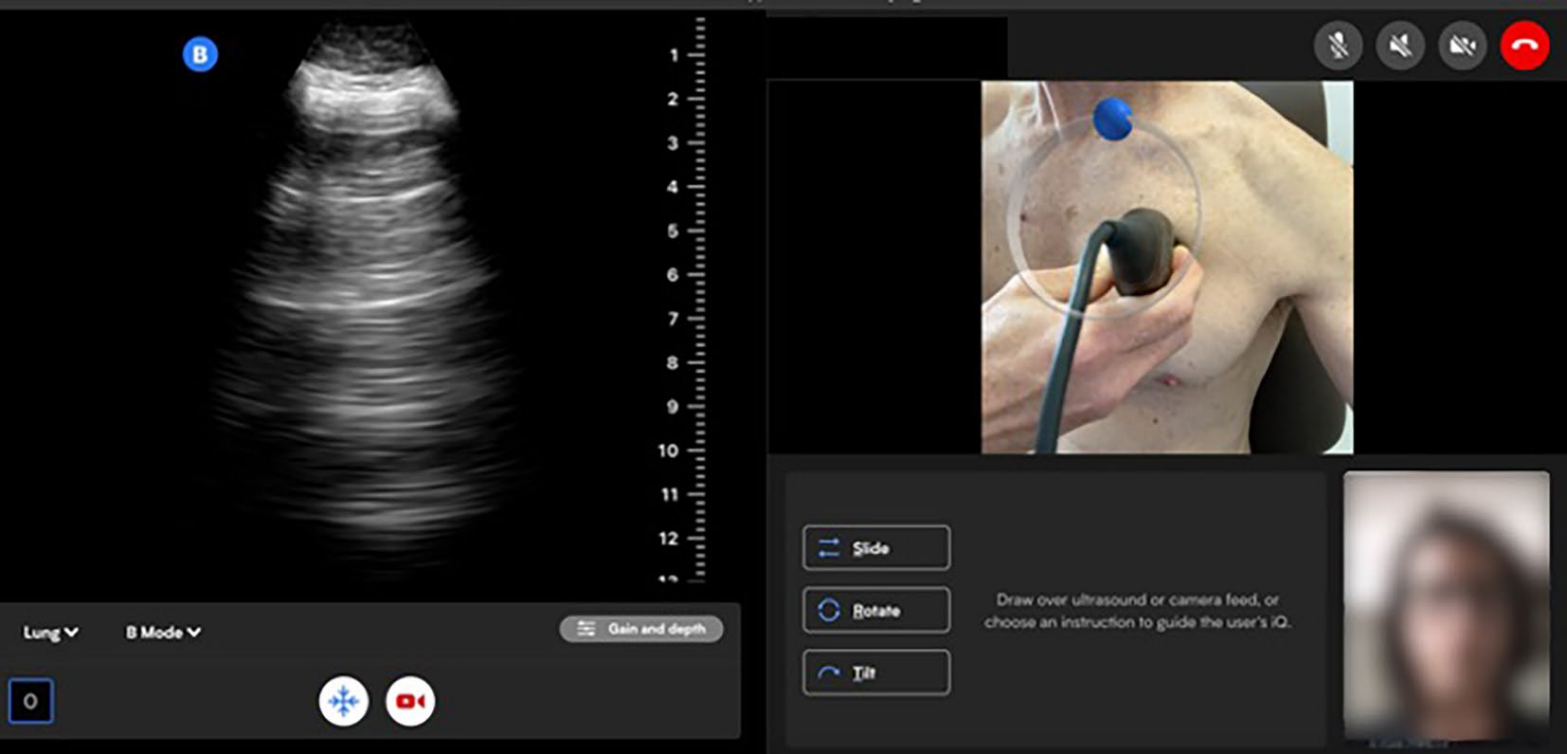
Example of a patient-performed remotely guided lung ultrasound session from the viewpoint of the guiding clinician
